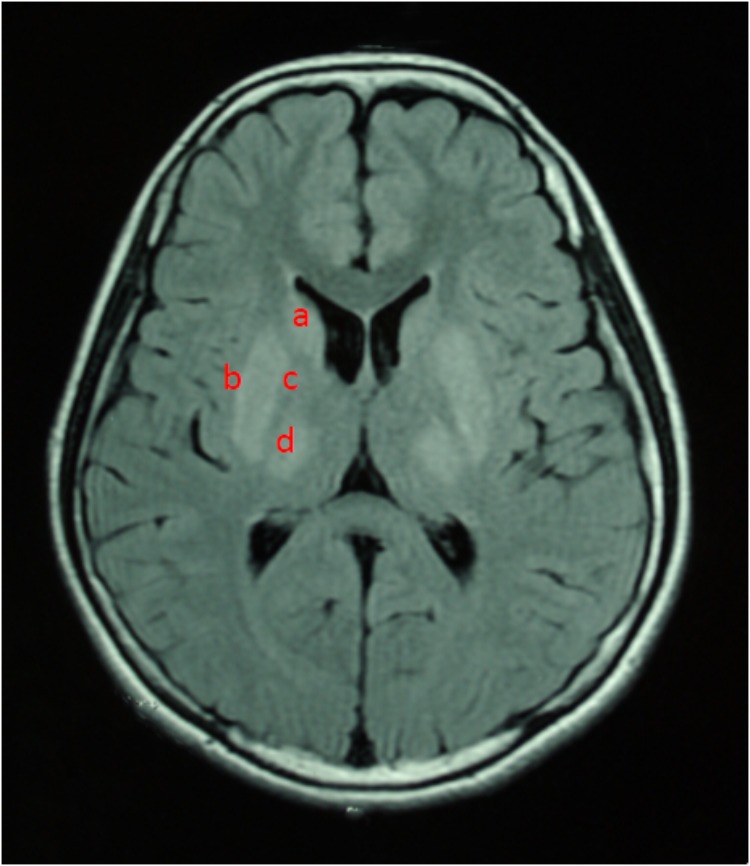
T2WI FLAIR image in a 12-year-old female. Axial images show hyperintensity in caudate nucleus (a), putamen (b), globus pallidus (c), and thalamus (d).
